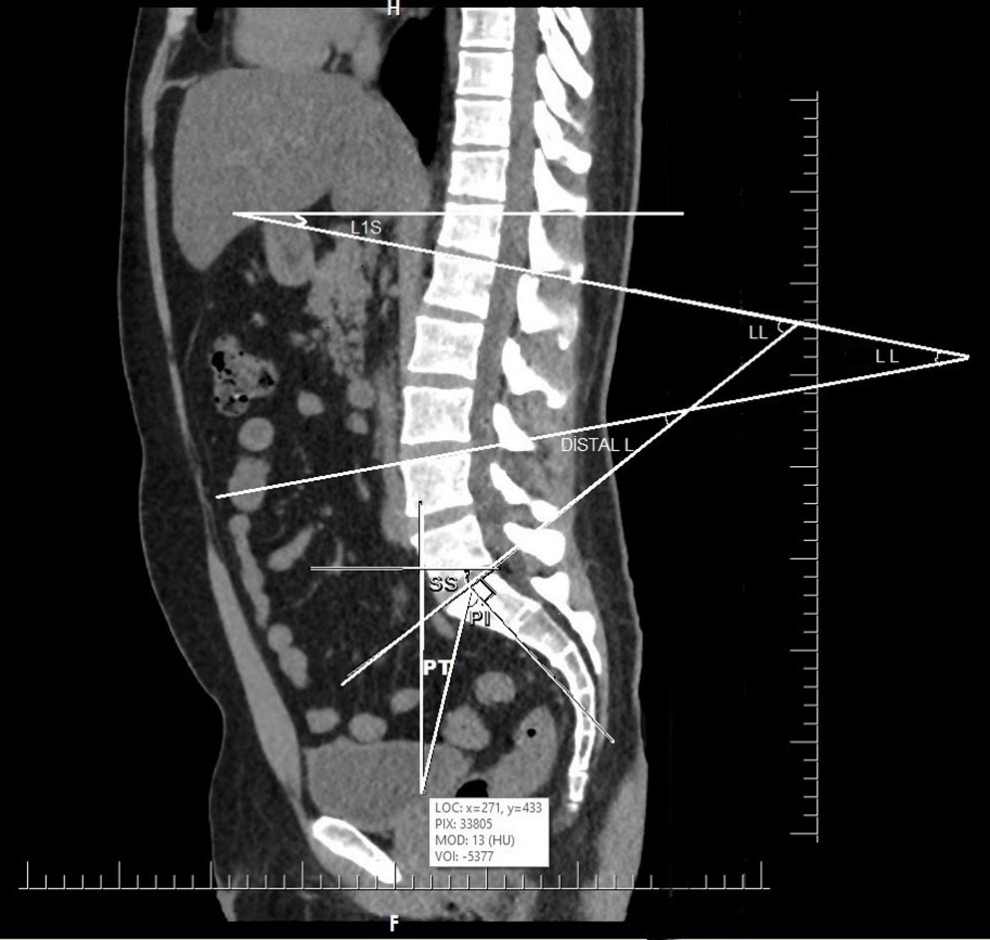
Spinopelvic parameters on a midsagittal slice of lumbosacral computed tomography. PI: Pelvic Incidence, PT: Pelvic Tilt, SS: Sacral Slope, L1S: L1 Slope, LL: Lumbar Lordosis, PLL: Proximal Lumbar Lordosis, DLL: Distal Lumbar Lordosis
